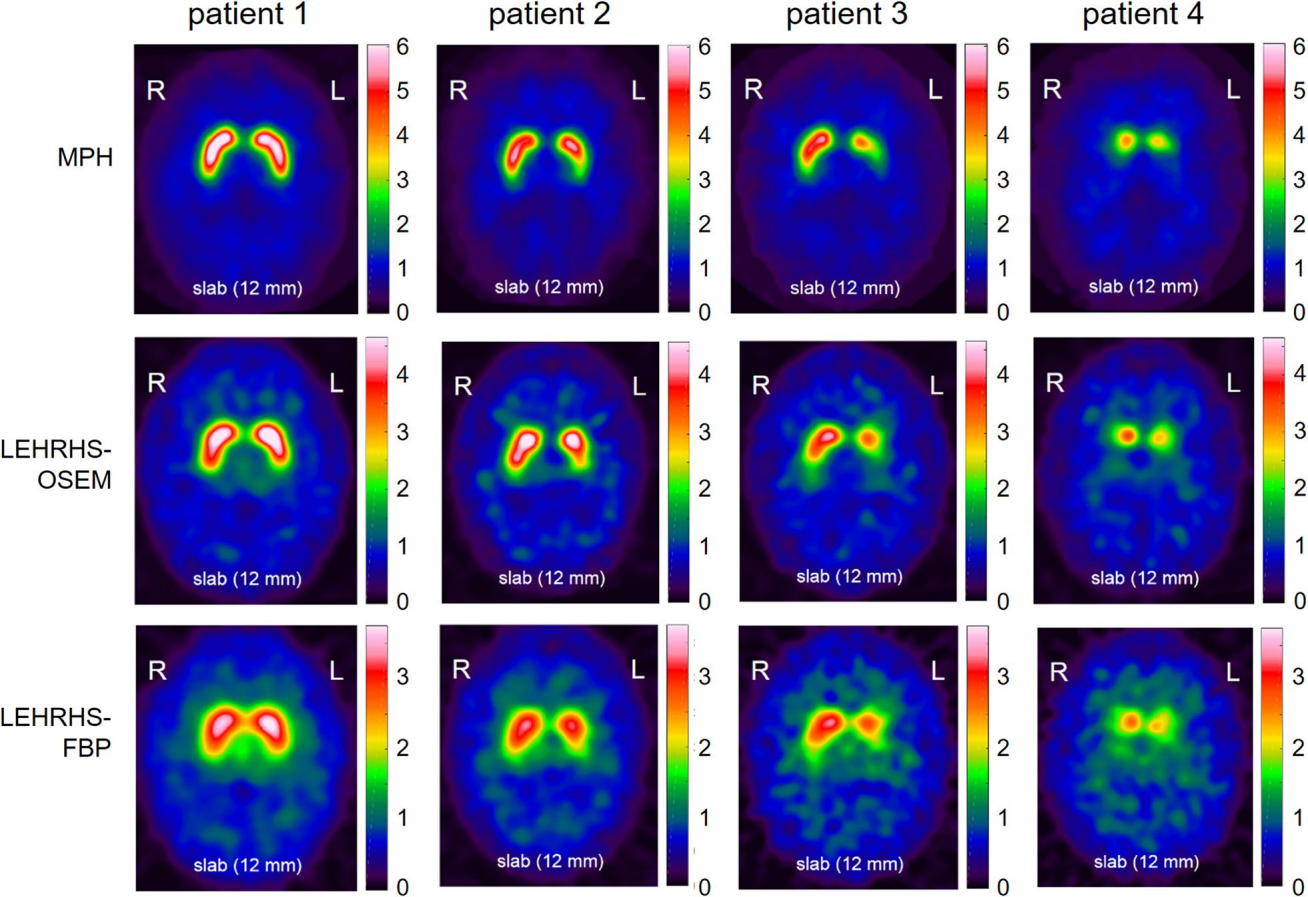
Representative DAT-SPECT images. Representative DAT-SPECT images from four different patients (columns) in the three different settings (rows)
